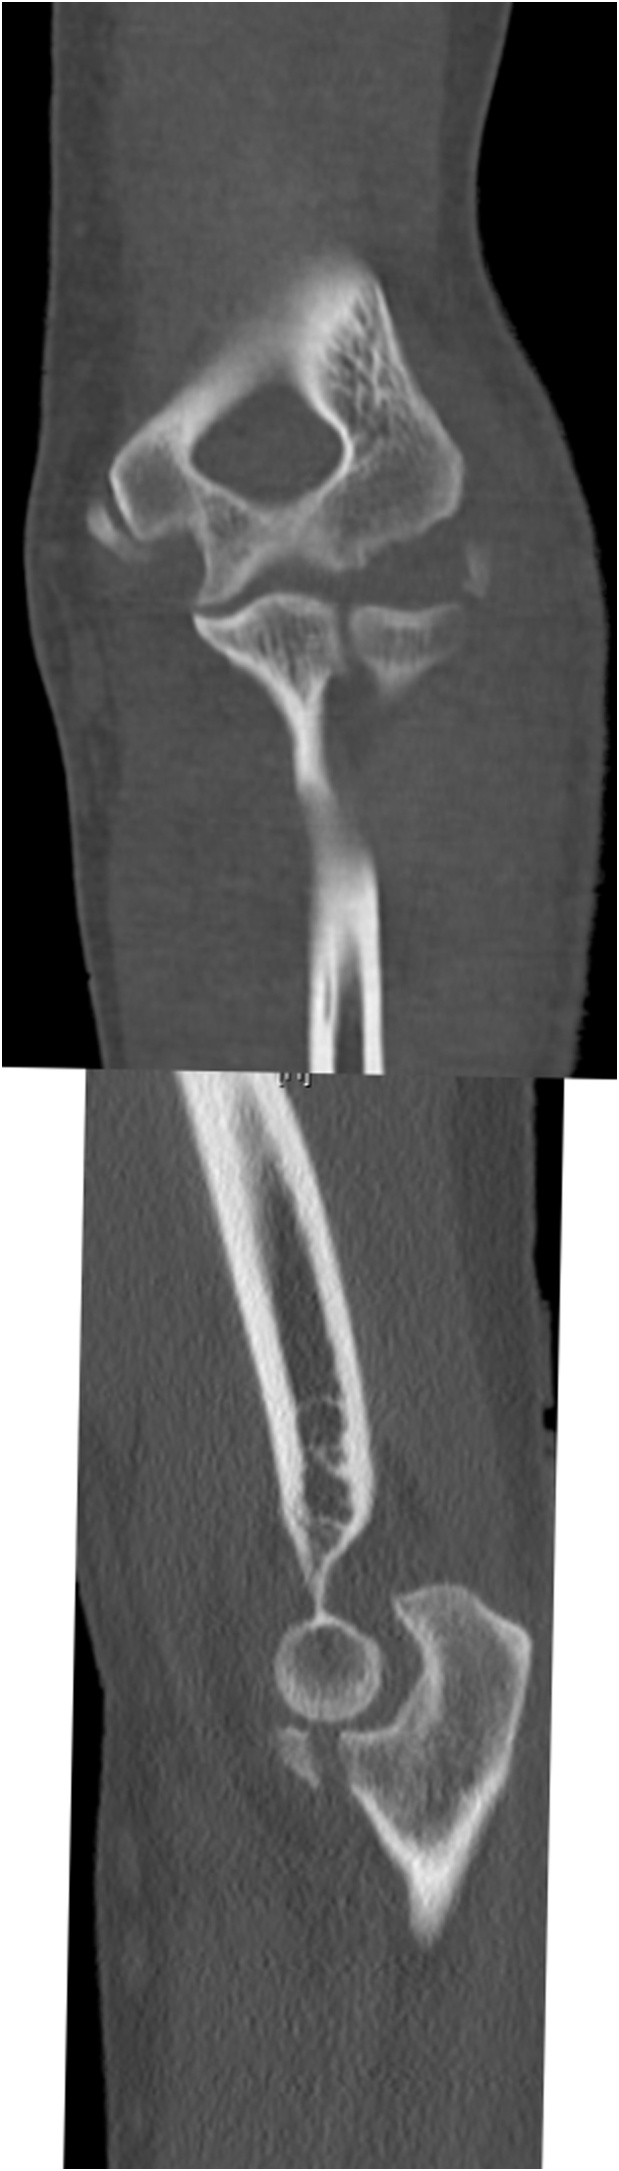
Coronal and sagittal CT scan of the left elbow showed avulsion fracture from the medial and lateral epicondyles of the distal humerus in addition to type 2 coronoid fracture.
